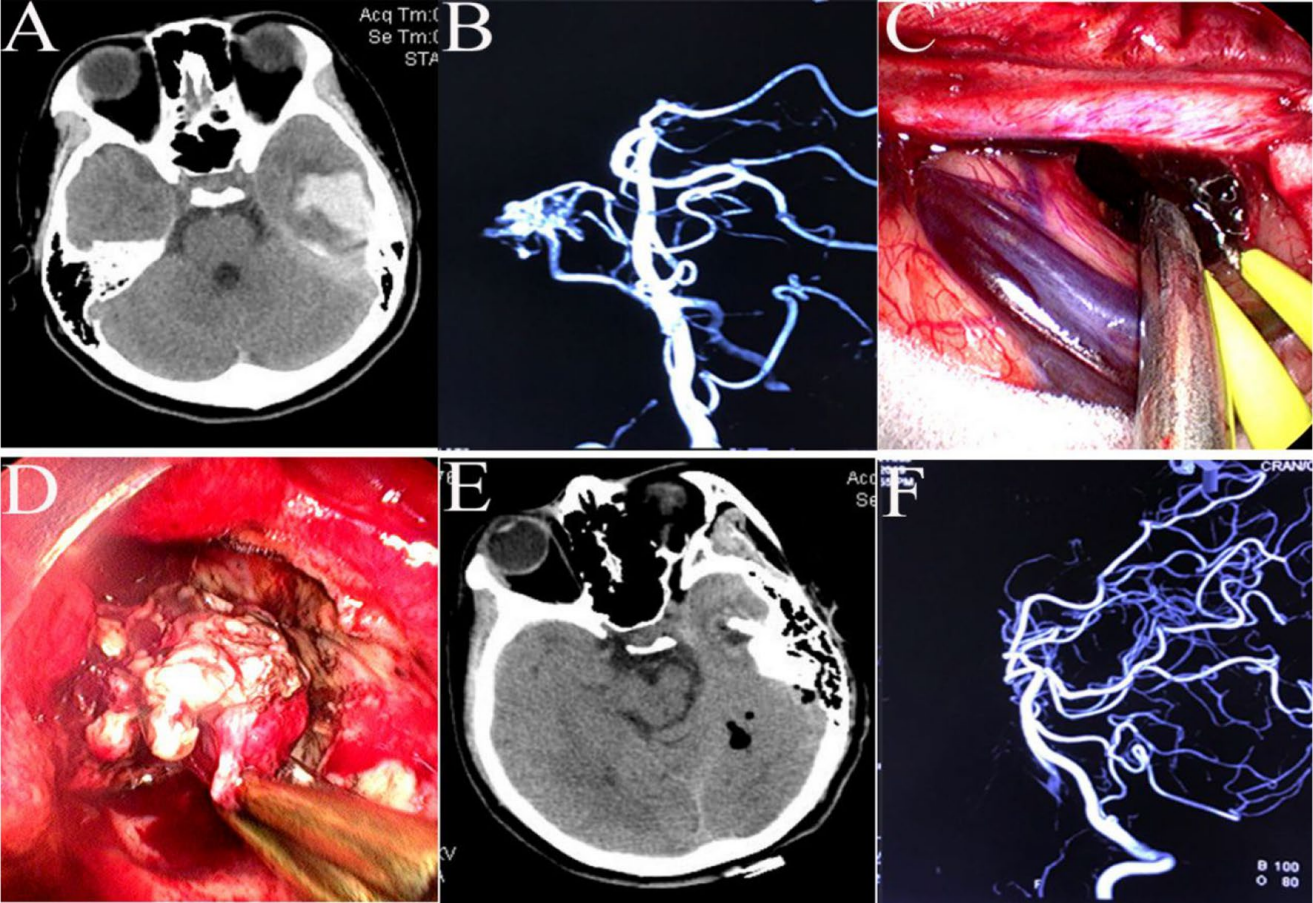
An AVM and hematoma were removed under neuroendoscopy. (A) CT showed a small hematoma at the back of the temporal lobe. (B) Digital subtraction angiography (DSA) showed a small AVM in the temporal lobe. (C) The hematoma was evacuated under neuroendoscopy. (D) The nidus of the AVM was circumferentially dissected under neuroendoscopy. (E) Postoperative CT showed complete removal of the hematoma. (F) Postoperative DSA showed no signs of the AVM.
